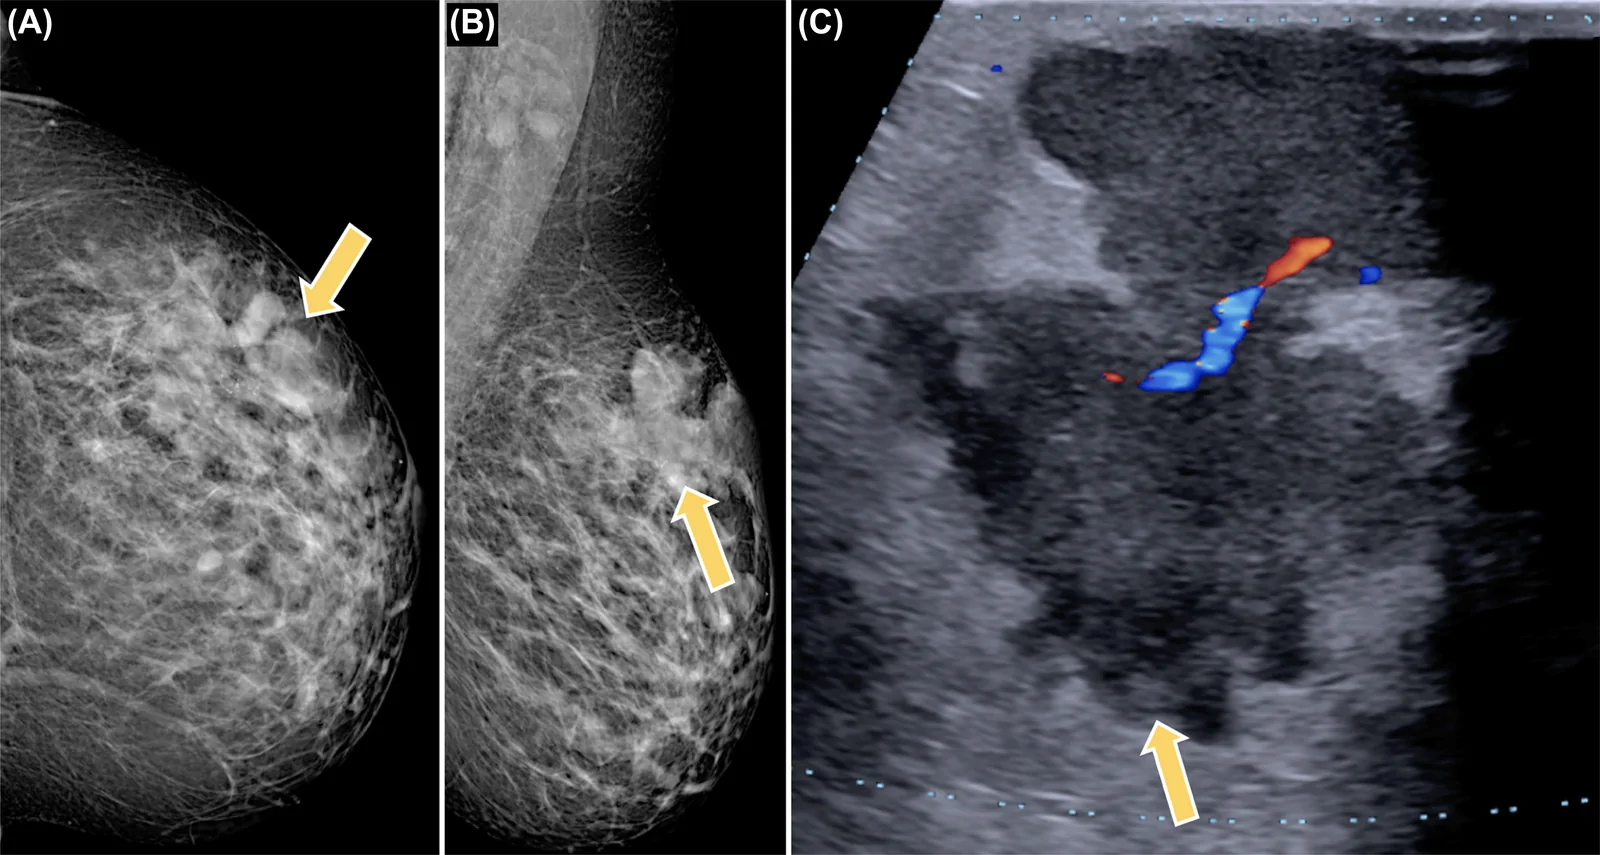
BI-RADS 4B lesion. Craniocaudal (A) and medio-lateral oblique (B) MMG views of the left breast show an irregular, microlobulated nodule located in the mid-depth of the upper outer quadrant (orange arrow), with associated architectural distortion. US (C) shows an irregular, non-parallel nodule with microlobulated margins and a central Doppler signal (orange arrow).
